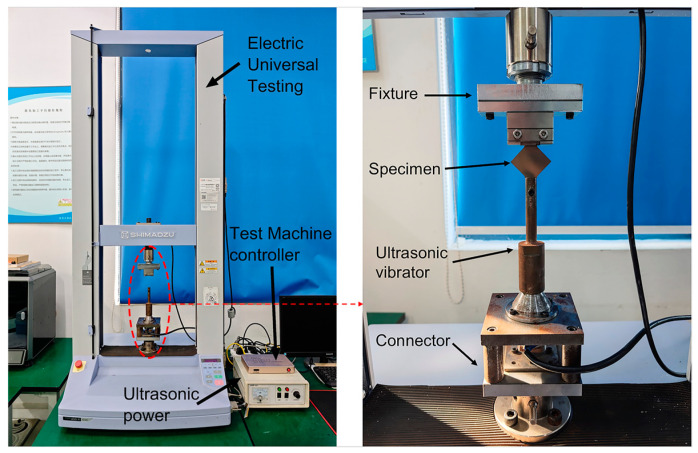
Ultrasonic-vibration-assisted tensile testing device.
